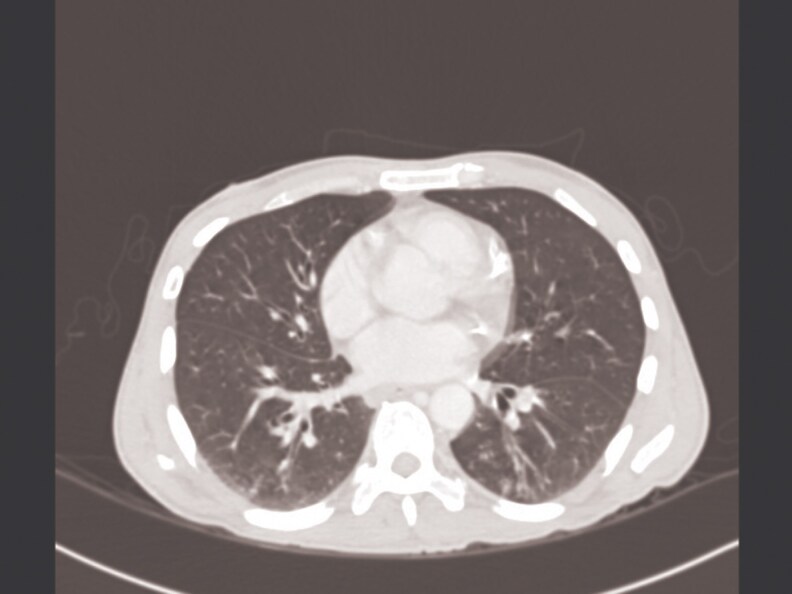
Radiological image (eg, CT scan) showing tree-in-bud pattern and nodules in a pulmonary *M riyadhense* infection (case 2; Table 2).
